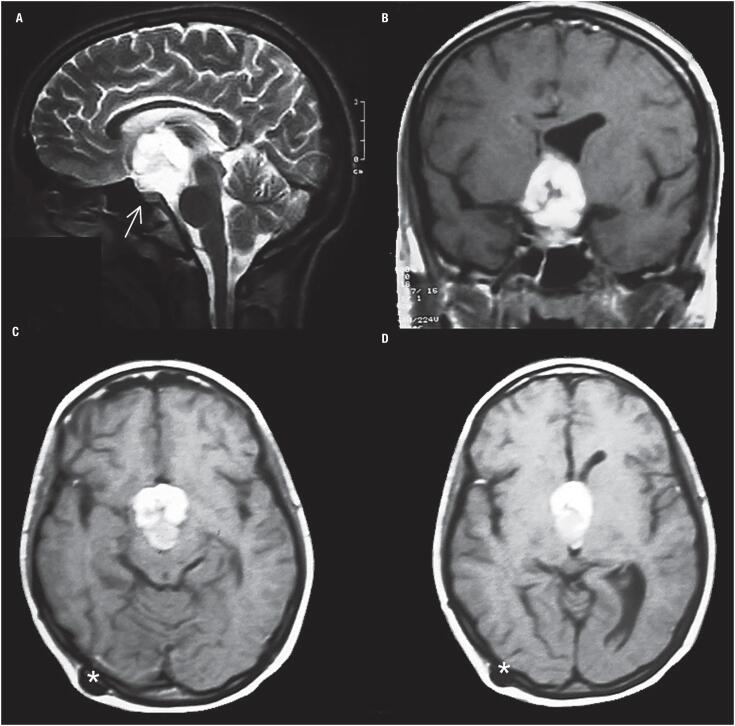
Patient 1. Magnetic resonance imaging findings: sagittal, coronal and axial images. A suprasellar ovoid mass with homogeneous enhancement after gadolinium administration is shown. (A) The sagittal T2 weighted image shows a suprasellar hyperintense ovoid mass with third ventricle extension. Note the normal pituitary gland in a normal sella (arrow). (B) The coronal T1 weighted image shows avid and homogeneous enhancement after gadolinium administration and ventricular asymmetry despite the septostomy and shunt. (C and D) The axial T1 weighted images with gadolinium show the involvement of the tumor in the interpeduncular cistern and the third ventricle. No hydrocephalus was detected due to the ventriculo-peritoneal shunt (asterisks).
